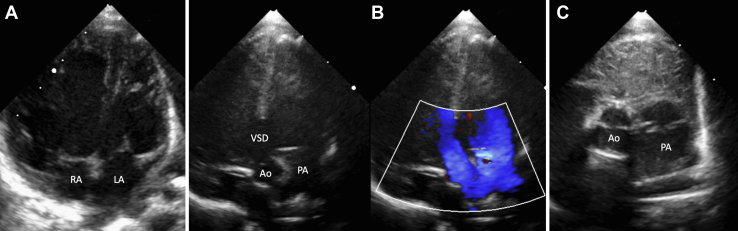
Echocardiography (A) The apical 4-chamber view. (B) The anterior apical 5-chamber view with and without color Doppler. (C) The parasternal short-axis view focused on great arteries. Ao = aorta; LA = left atrium; PA = pulmonary artery; RA = right atrium; VSD = ventricular septal defect.
